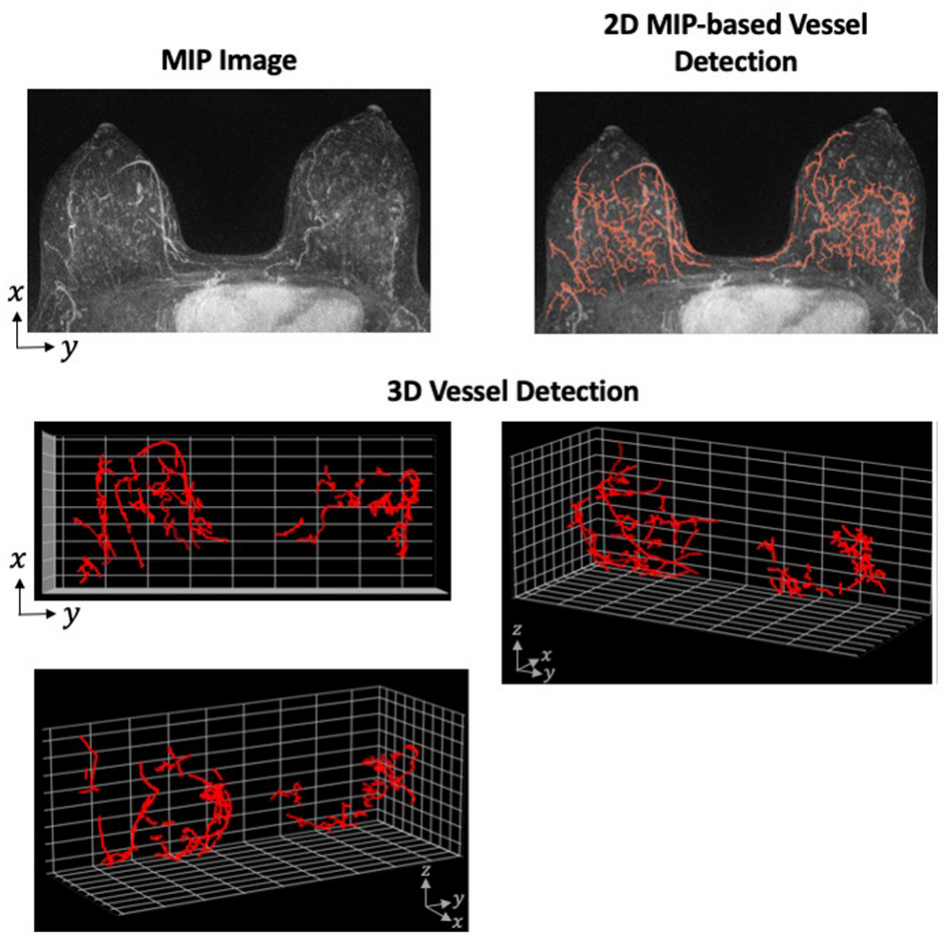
Vessel Detection Performed in 2- and 3-Dimensions. A Hessian-based approach was applied to both 2D MIP and full 3D MRI using a single sample from our data set. The vessel detection algorithm was based on a combination of methods described by Vignati et al. (130) and Wu et al. (48) with parameters determined using our data set. The MIP images shows many filamentary-like enhancing structures at the base of the breast. These structures were consequently detected as vessels in the 2D approach. However, the distance between these structures on the z-axis are not preserved when projected to 2-dimensions. When detecting vessels using the full 3D image, these enhancing structures prove to be unconnected and are, therefore, not detected as vessels.
